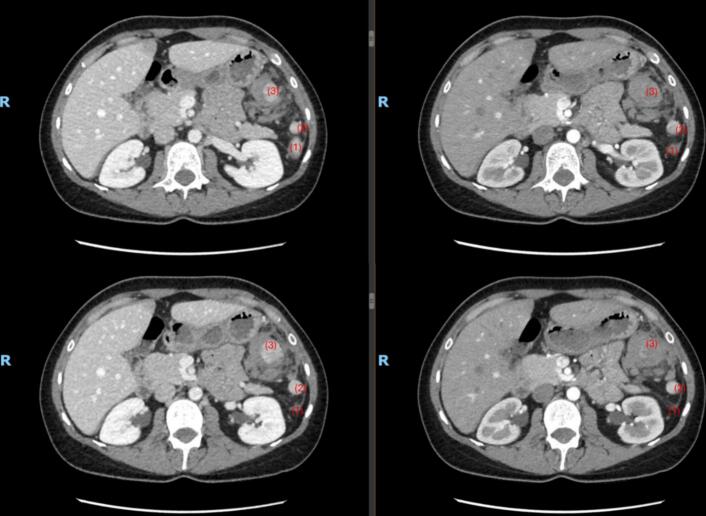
Axial cuts of the abdominal CT scan with contrast injection done upon presentation: portal phase on the left side and arterial phase on the right side. (1) Represent the caudal main spleen, (2) represent the unremarkable accessory spleen, (3) represent the ruptured accessory spleen with surrounding pooling hematoma.
